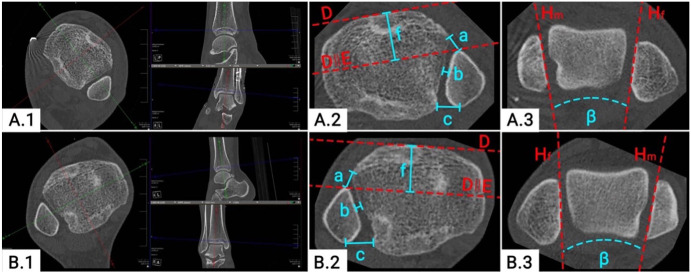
Quality of DTFJ reduction on bilateral CT images. (A.1) DTFJ reconstruction of the reduced ankle. (A.2) Measurement of the DTFJ reduction of the reduced ankle. (A.3) NTDA of the reduced ankle. (B.1) DTFJ- reconstruction of the contralateral side. (B.2) Measurement of the DTFJ reduction of the contralateral ankle. (B.3) NTDA of the contralateral side. a, anterior tibiofibular distance; b, central tibiofibular distance; β, NTDA; c, posterior tibiofibular distance; CT, computed tomographic; D, tangent to the anterior aspect of the tibia; DTFJ, distal tibiofibular joint; E, translation of D to the most anterior part of the fibula; f, sagittal DTFJ-translation of the reduced ankle; H, tangent to the medial malleolus (m) or fibula (f); NTDA, Nault talar dome angle; ||, translation.
